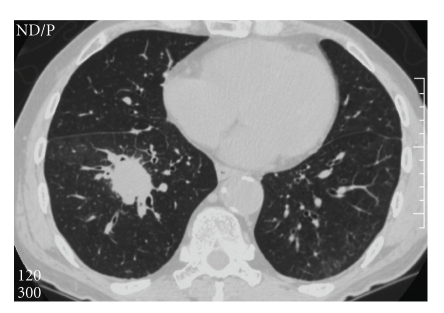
Computed tomography of the thorax showed a soft tissue density nodule with spiculated margins and vascular convergence in the lower lobe, measuring 3 × 2 cm, in the right lung.
